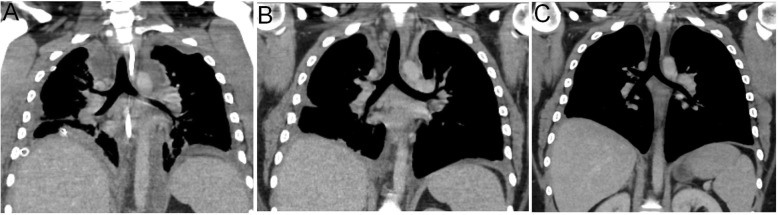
(a) Day 7 CT scan- acme of the mediastinal infiltration, with abscesses formation (3 days after surgery); (b) CT scan at M1 after intensive care unit admission; (c) CT scan at M2 after intensive care unit admission
